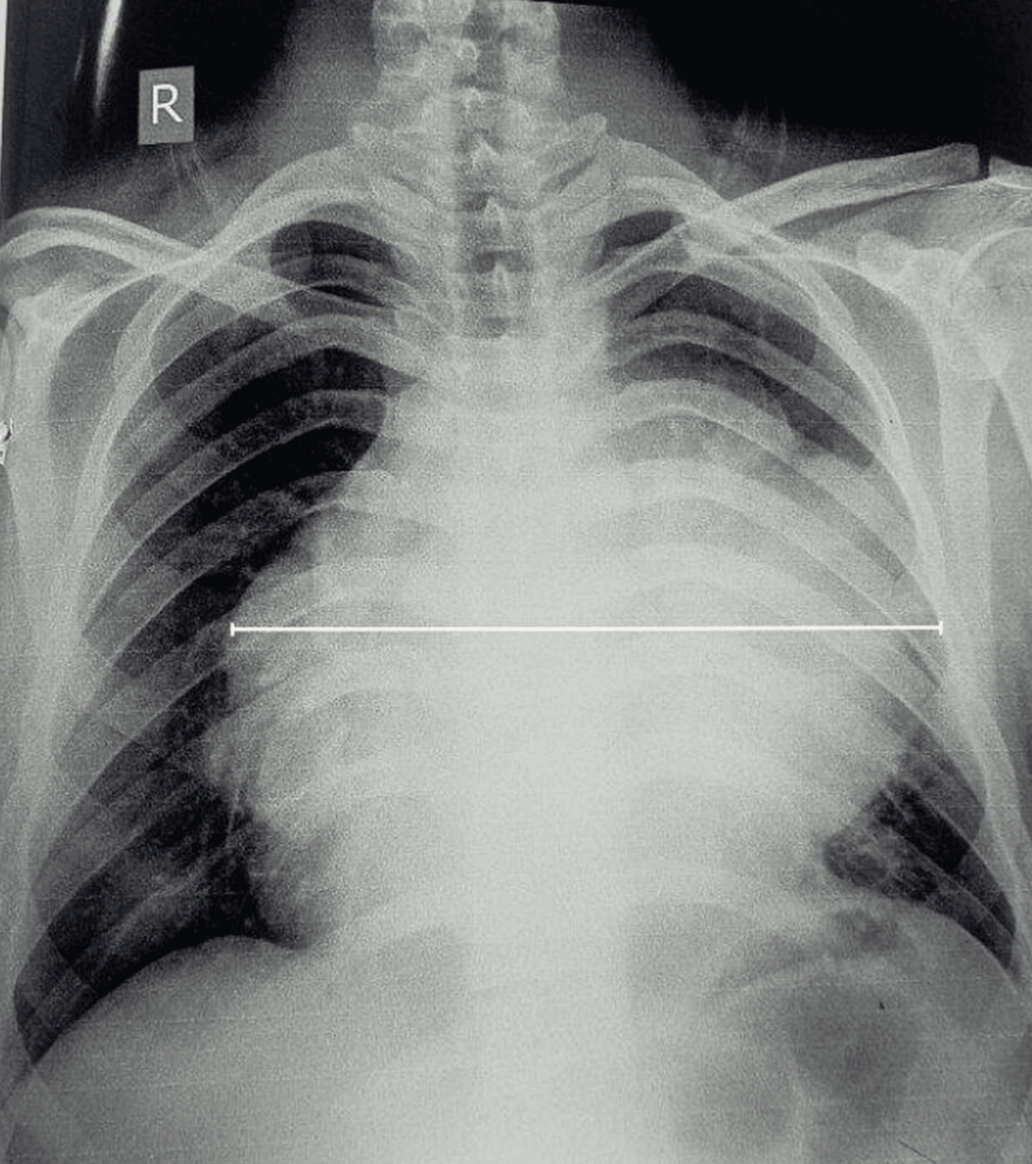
Preoperative chest X-ray showing large homogenous opacity in the mediastinum SICU: surgical intensive care unit
